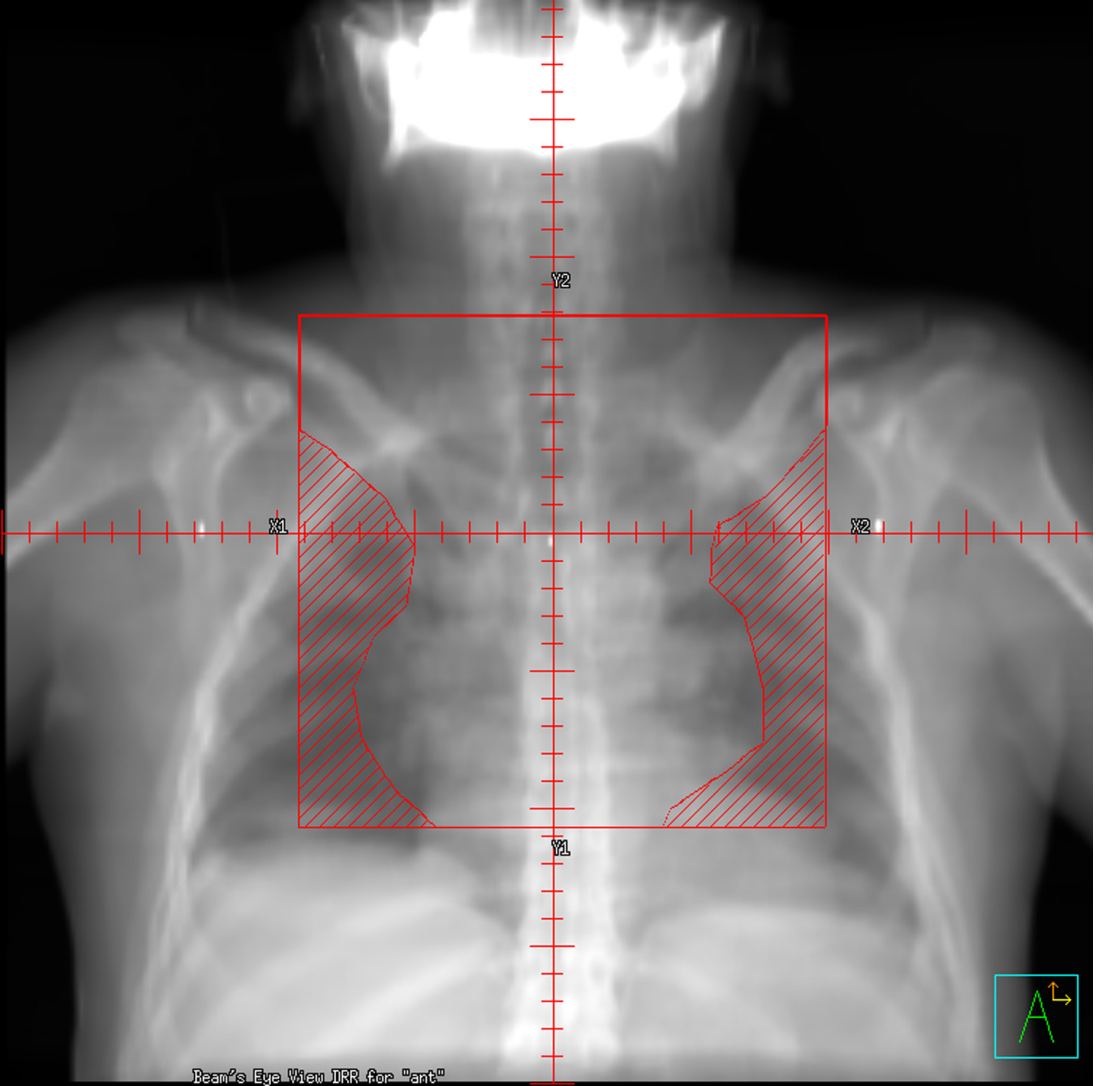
Digitally reconstructed radiographs demonstrating: mediastinal involved field RT (IFRT).
